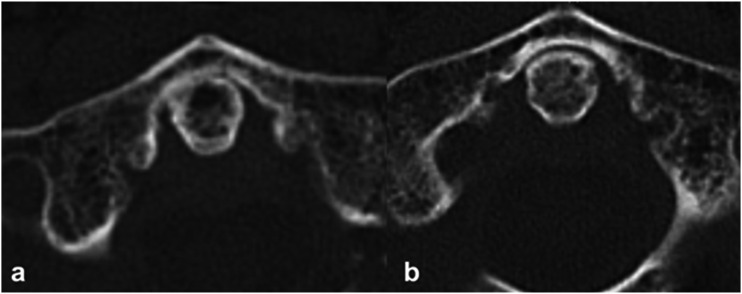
Axial computed tomography reconstruction showing (a) severe degeneration of the atlanto-odontoid joint in patients with low-energy trauma and (b) absence of degeneration in patients with high-energy trauma.
